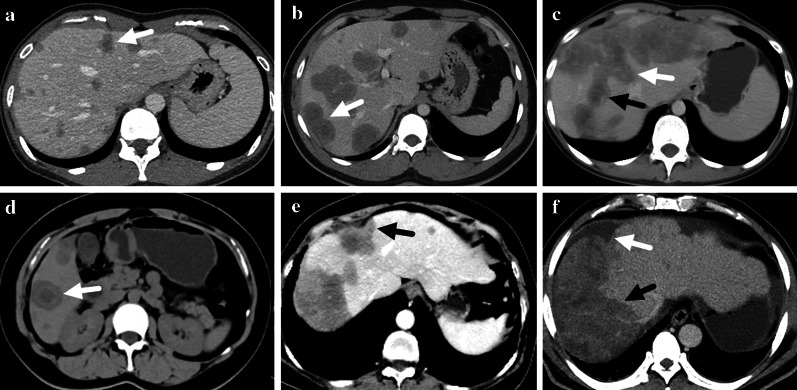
a A nodular lesion with target sign was marked with a white arrow. b A locally coalescent lesion with target sign was marked with a white arrow. c A diffusely coalescent lesion occupied multiple liver segments (marked with a white arrow). Target sign could also be found in the lesion (marked with a black arrow). d Target sign could be found on unenhanced CT scan (marked with a white arrow). f Capsular retraction caused by a subcapsular lesion could be detected (marked with a black arrow). e A diffusely coalescent lesion (marked with a black arrow) was accompanied with capsular retraction and perihepatic effusion (marked with a white arrow)
